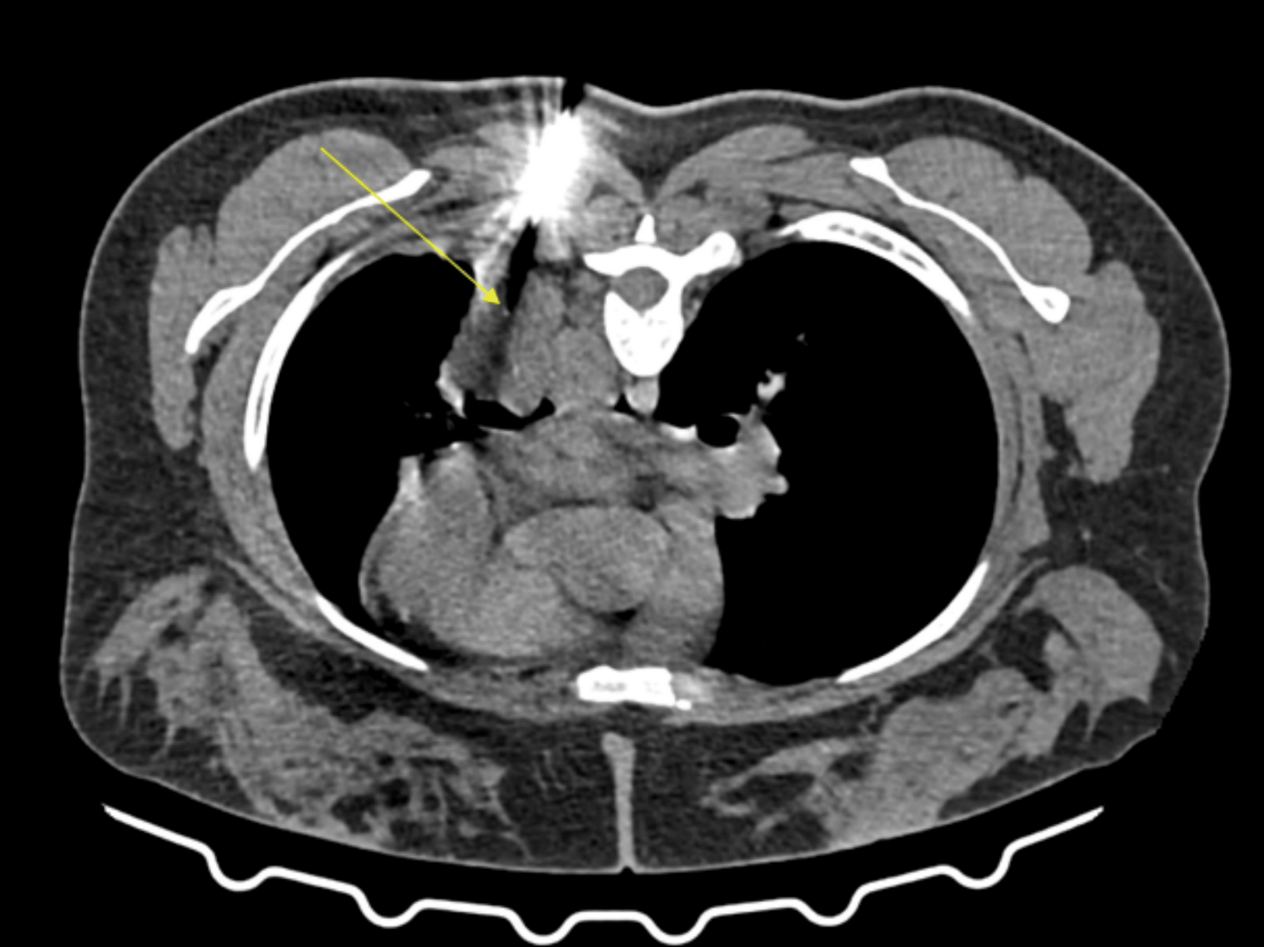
Computed tomography (CT)-guided biopsy performed in the prone position from the left lower lobe lesion shows the precise placement of the tip of the biopsy needle (yellow arrow), highlighting the technical precision.
